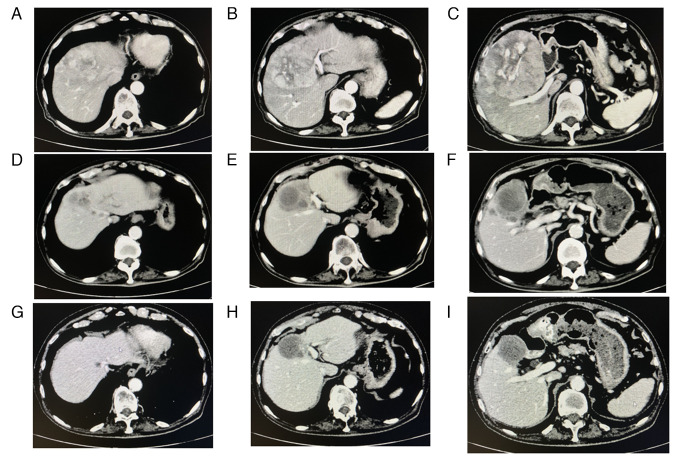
Changes in abdominal CT scan images obtained during combination therapy with atezolizumab plus bevacizumab. (A-C) Prior to therapy, a CT scan demonstrated multiple hepatocellular carcinomas in the liver and swelling of the lymph nodes in the paraaortic lesion and other sites. (D-F) After 5 months of combination therapy, a CT scan demonstrated the disappearance of any intratumoral arterial enhancement in any of the target lesions. (G-I) At 4 months after the combination treatment was suspended, a CT scan did not demonstrate any recurrences. CT, computed tomography.
